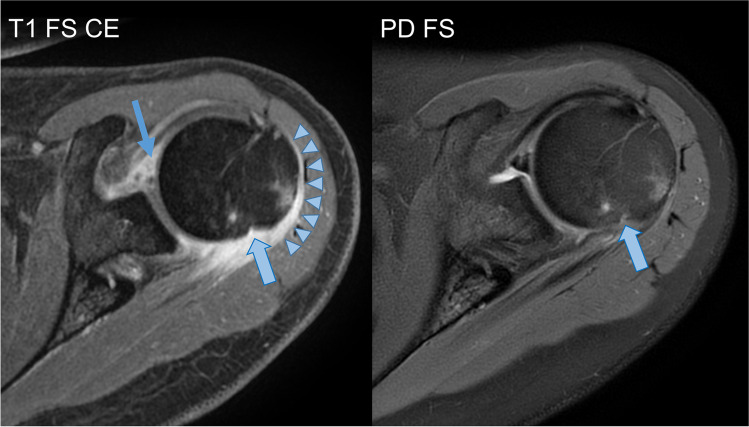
Capsulitis, synovitis and small erosion of the greater tuberosity in a 26-year-old female—capsulitis (arrow heads) and synovitis (solid arrow) identified as diffuse contrast enhancement, small erosion of the greater tuberosity (filled arrow), note how capsulitis and synovitis are better appreciated on T1-weighted fat-saturated (FS) contrast-enhanced (CE) MRI, with only little oedema on the PD-weighted FS image
